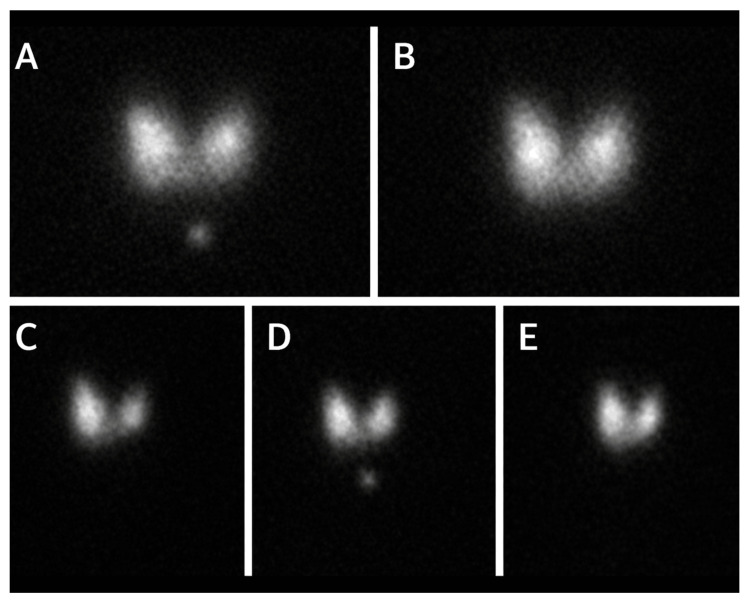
Radioactive iodine uptake and scan demonstrating diffusely increased tracer activity consistent with Graves' disease (A) Anterior thyroid scintigraphy image showing homogeneous radiotracer distribution throughout both lobes. (B) Additional anterior projection confirming uniform tracer uptake without focal hyperfunctioning or nonfunctioning nodules. (C) Right anterior oblique view demonstrating symmetric uptake within the right lobe. (D) Left anterior oblique view showing comparable uniform activity in the left lobe. (E) Supplemental projection confirming absence of focal defects or asymmetric radiotracer accumulation.
